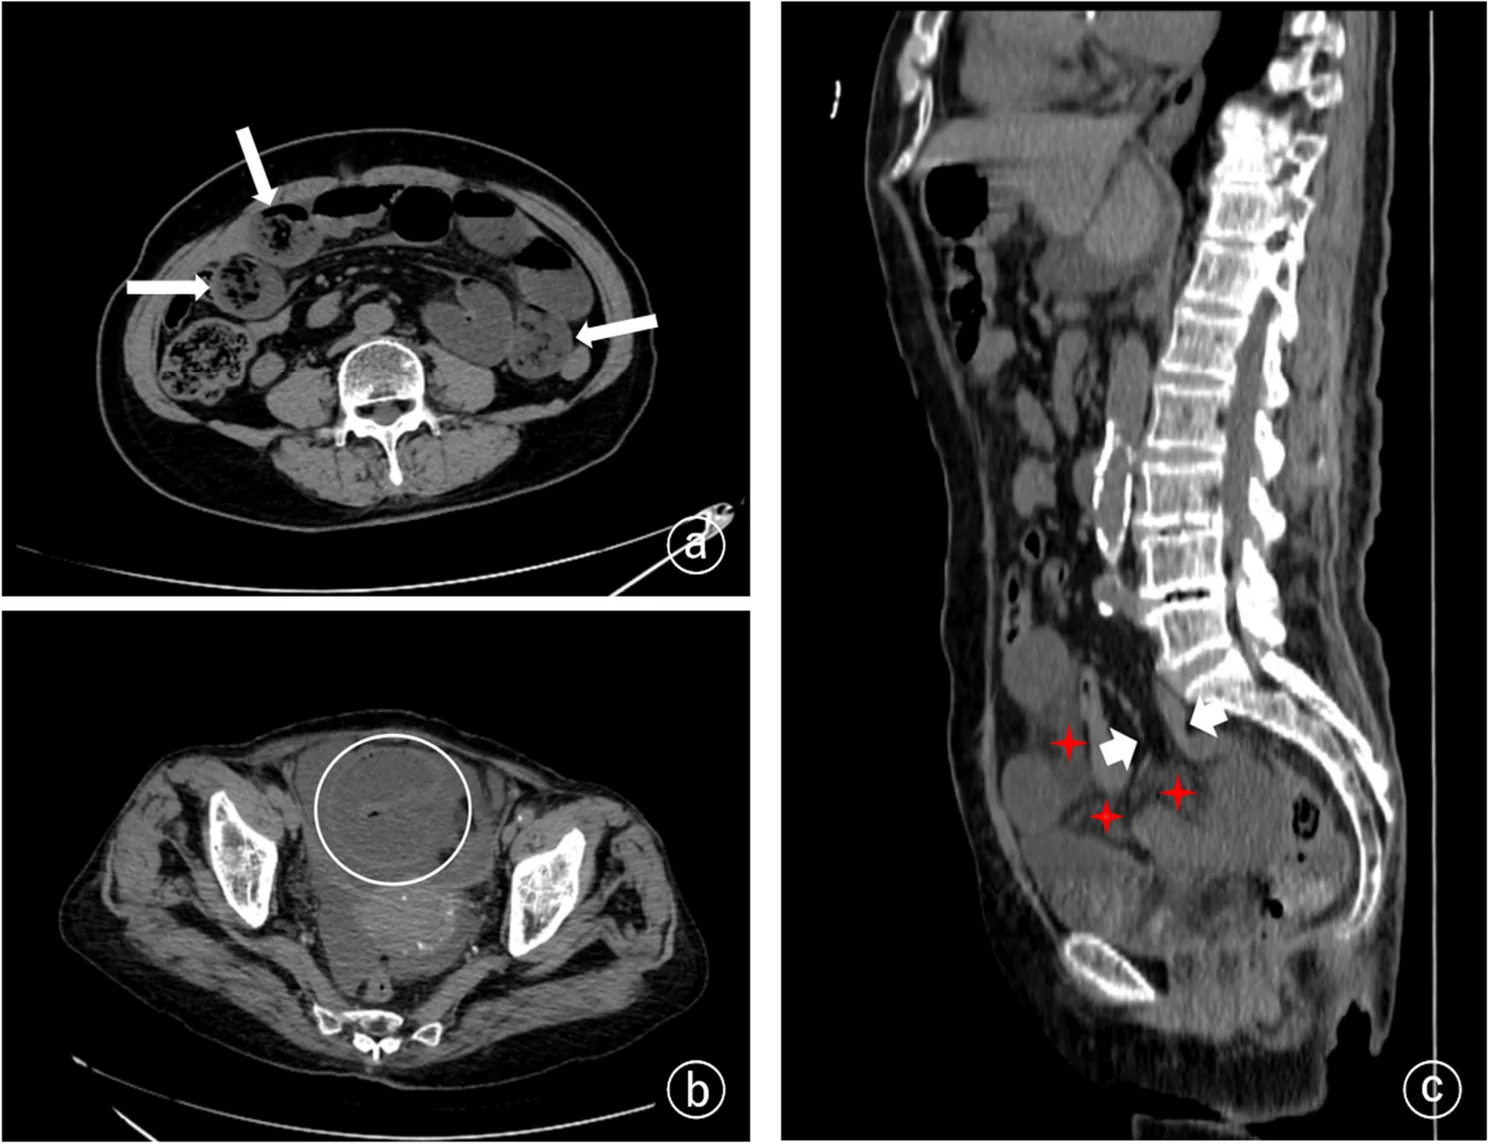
Characteristic CT signs of strangulated small bowel obstruction a small bowel feces sign (thick white arrow) ; b coffee bean sign (white circle) ; c mesenteric edema (red four-pointed star) and hernia orifice (thick white arrow)
